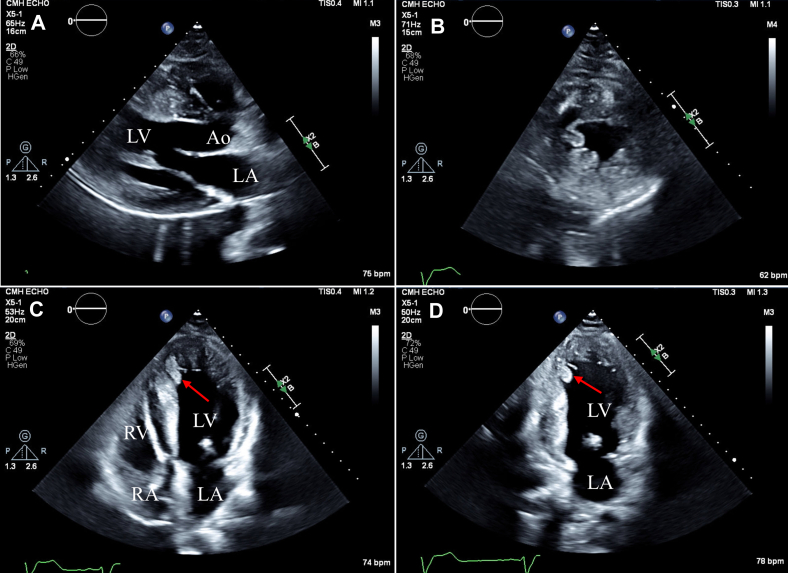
Progressive Features of Noncompaction With Apical Thrombus The patient's echocardiogram at the time of return to care at age 25. The parasternal long-axis view in diastole demonstrates LV cavity dilatation (A). The parasternal short-axis view demonstrates persistent evidence of noncompaction pathology (B). Apical 4- (C) and 2-chamber (D) views demonstrate apical dilation with a focal apical thrombus (red arrows). Ao = aorta; LA = left atrium; LV = left ventricle; RA = left atrium; RV = right ventricle.
